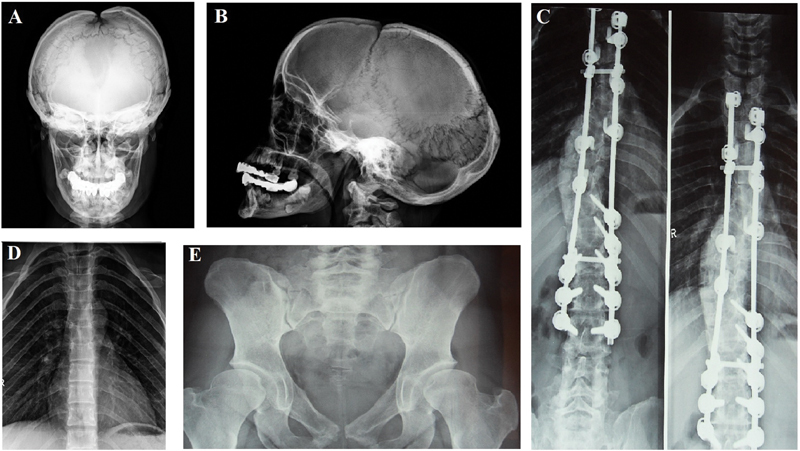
X-ray findings of the patients: (
